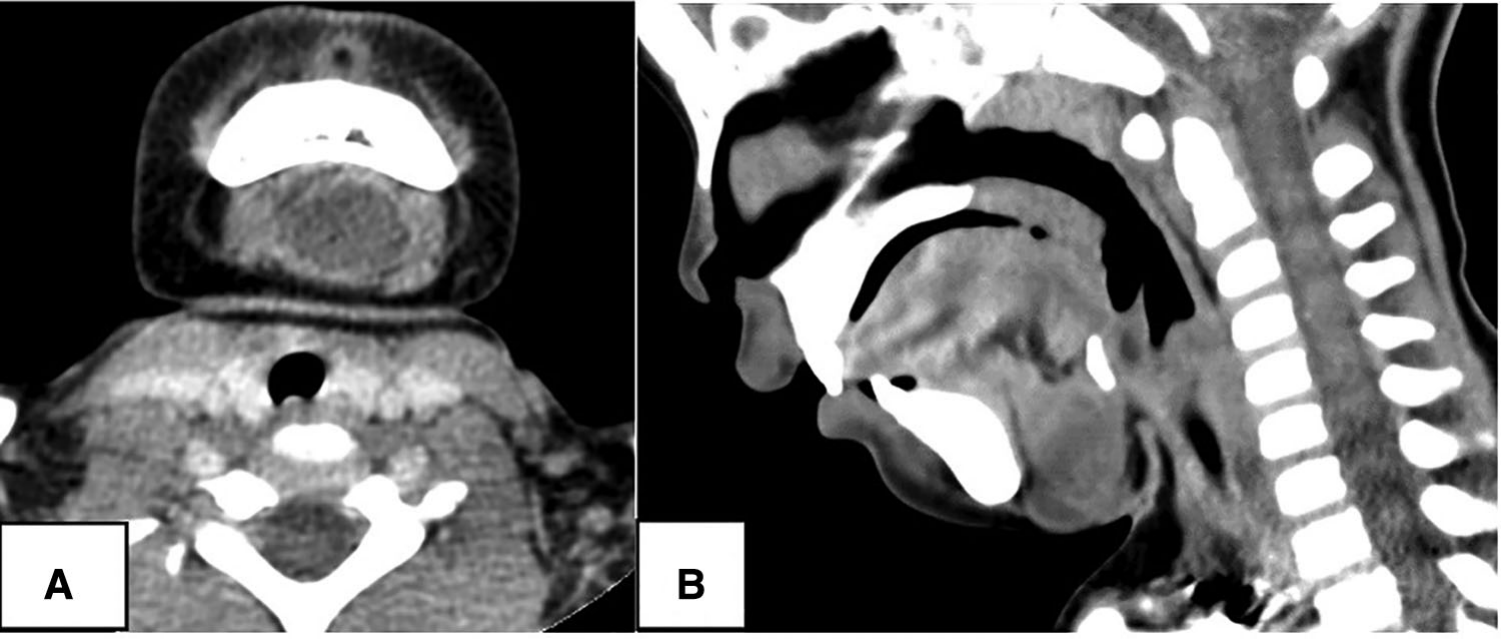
A 1.8-year-old female. CT showed a 2.1-cm sized cystic lesion in the midline neck connecting with hyoid bone (red circle), and misdiagnosed as thyroglossal duct cyst: (A) contrast-enhanced axial scan; (B) sagittal scan.
